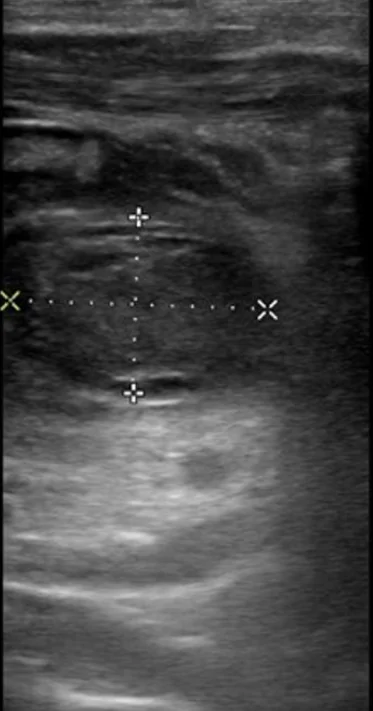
Ultrasound measurement of the internal jugular vein demonstrating a 1.22 × 1.80 centimeters intraluminal hyperechoic thrombus in a 58-year-old woman who presented to the emergency department with two days of progressively worsening right-sided neck pain and swelling.
